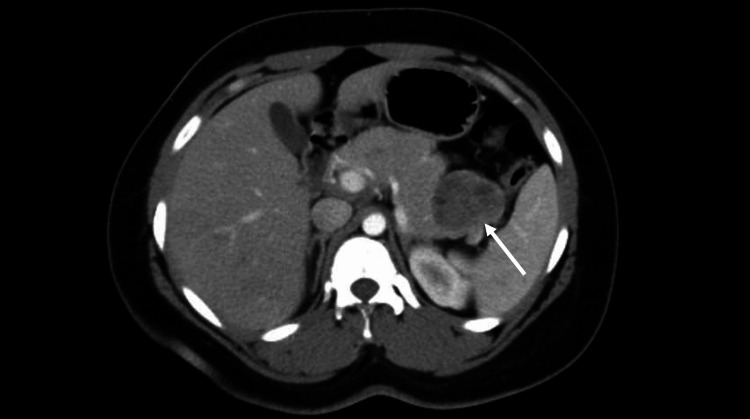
CT abdomen and pelvis with contrast exhibiting a 3.3 cm pancreatic mass adjacent to splenic hilum (white arrow) CT: computed tomography
